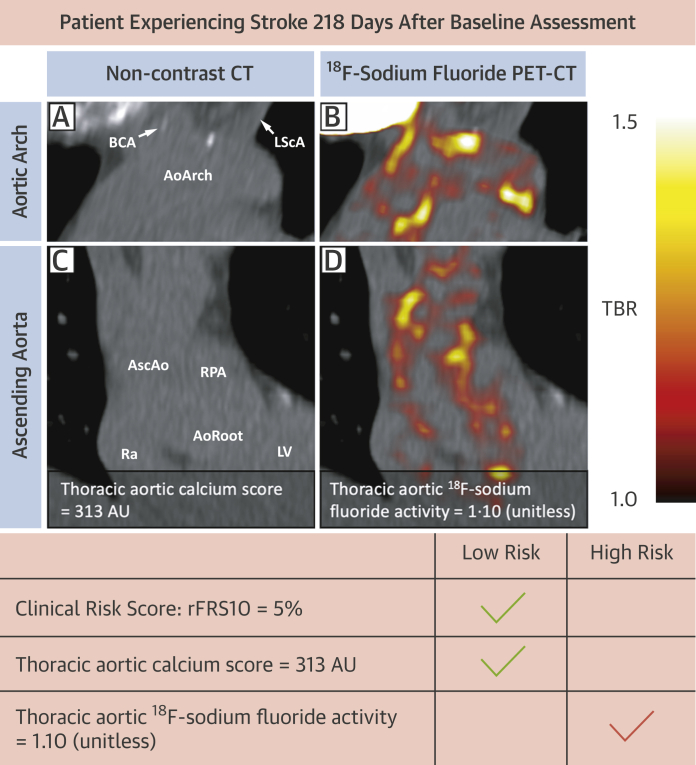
Example of Association Between ^18^F-Sodium Fluoride Positron Emission Tomography and Future Stroke Baseline noncontrast computed tomography (CT) and ^18^F-sodium fluoride positron emission tomography (PET) of the aortic arch (A and B) and ascending aorta (C and D). A 60-year-old patient with hypertension and moderate aortic stenosis, on aspirin and clopidogrel. Overall low clinical risk score and thoracic aortic calcium scores, but high thoracic aortic ^18^F-sodium fluoride activity. Patient had bilateral posterior circulation infarcts at day 218 of follow-up with normal carotid arteries and no atrial fibrillation. AoArch = aortic arch; AoRoot = aortic root; AscAo = ascending aorta; AU = Agatston units; BCA = brachiocephalic artery; LScA = left subclavian artery; LV = left ventricle; Ra = right atrium; rFRS10 = Revised 10-year Framingham Stroke Risk Score; RPA = right pulmonary artery; other abbreviation as in Figure 1.
